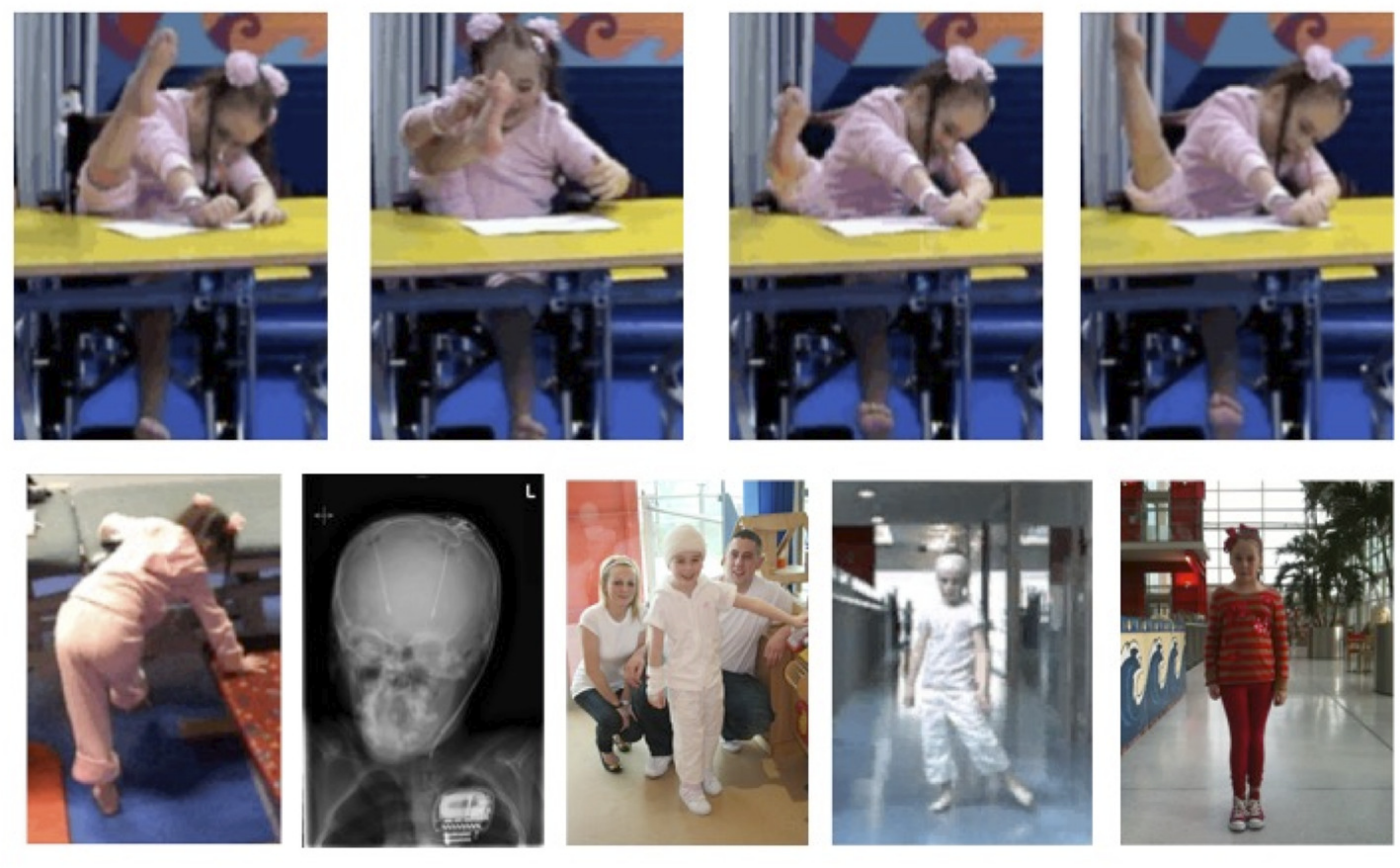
DYT-1 dystonia and intrusive “ballerina postures” and improvements with Deep Brain Stimulation (DBS). Video frames over time before and after DBS. Onset age 6.0 years with rapid progression to wheel-chair mobility by age 6.5 years. (Top) “Ballerina posturing” of the right leg interferes with lying and sitting (see also Figure 1C). [Lower (left-right)]: unable to stand or walk; head and chest X-ray post ACTIVA RC rechargeable DBS implant, age 7 years; standing unsupported within days of DBS; walking unsupported 3 months post DBS with a left circumducting gait; fully recovered at 3 years post-DBS. *Courtesy J-P Lin*.
